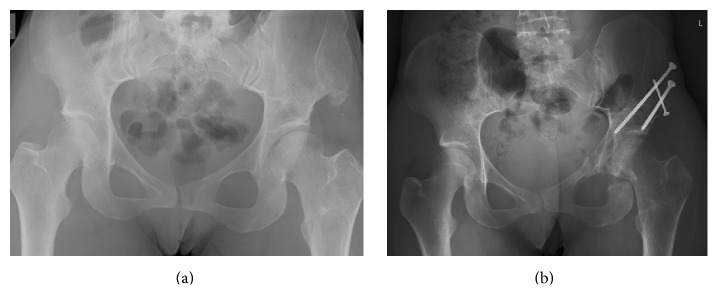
(a) AP pelvic X-ray demonstrating acetabular dysplasia of the left hip. (b) Normalisation of the CEA and healing of the osteotomy sites 3 months after periacetabular osteotomy.
